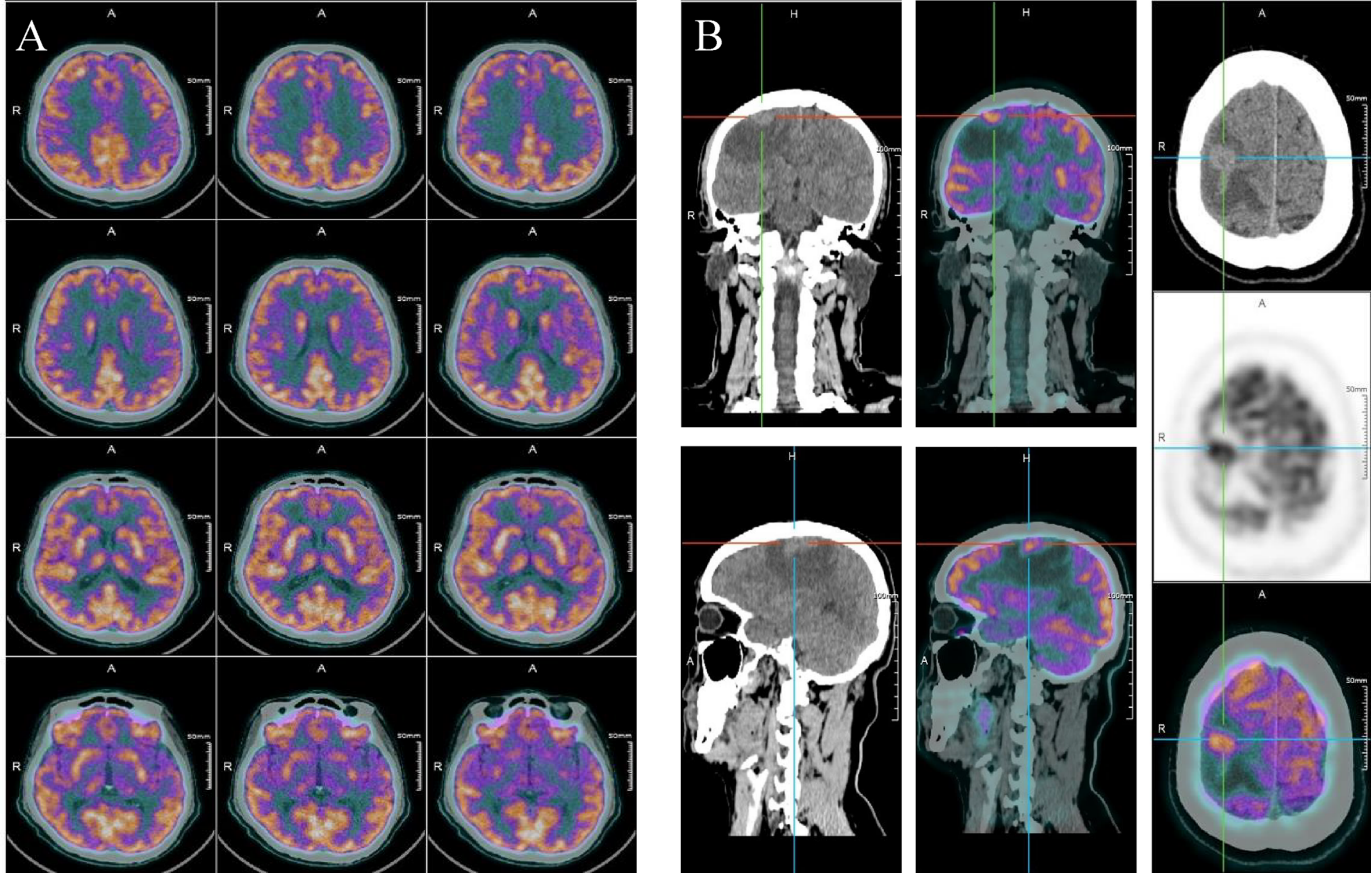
(A) PET-CT scan of the brain before liver surgery. (B) PET-CT scan of the brain 3 months after liver surgery.
